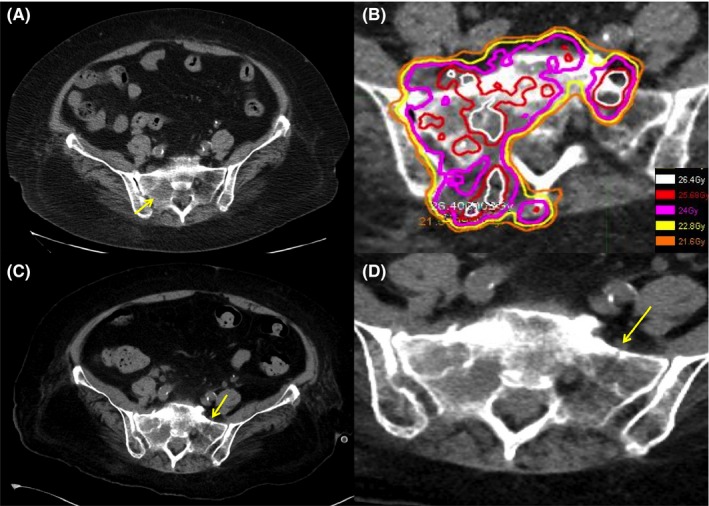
A, Axial CT scan slices. The tumor was located in the right sacral bone extending along the right sacral foramina (yellow arrow). B, Axial slices showing representative dose distribution for carcinoma. C and D, Axial slices showing the fracture line in the left sacral alae (yellow arrow)
